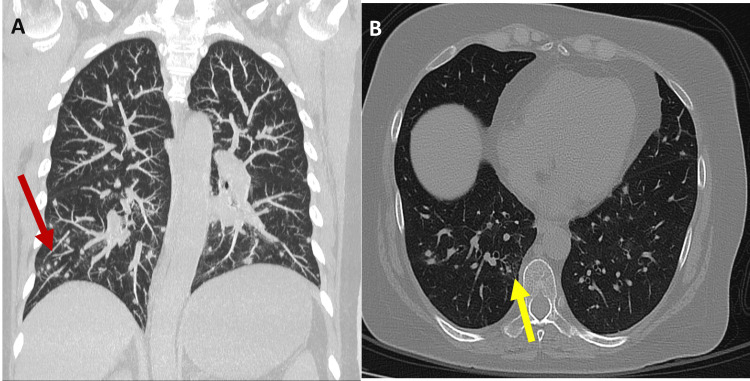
Chest CT in A) coronal plane MIP (maximum intensity projection) that shows several bilateral and diffuse solid nodules (red arrow), and B) axial plane MPR (multiplanar reformation) shows ground-glass pattern and atelectatic bands (yellow arrow).
